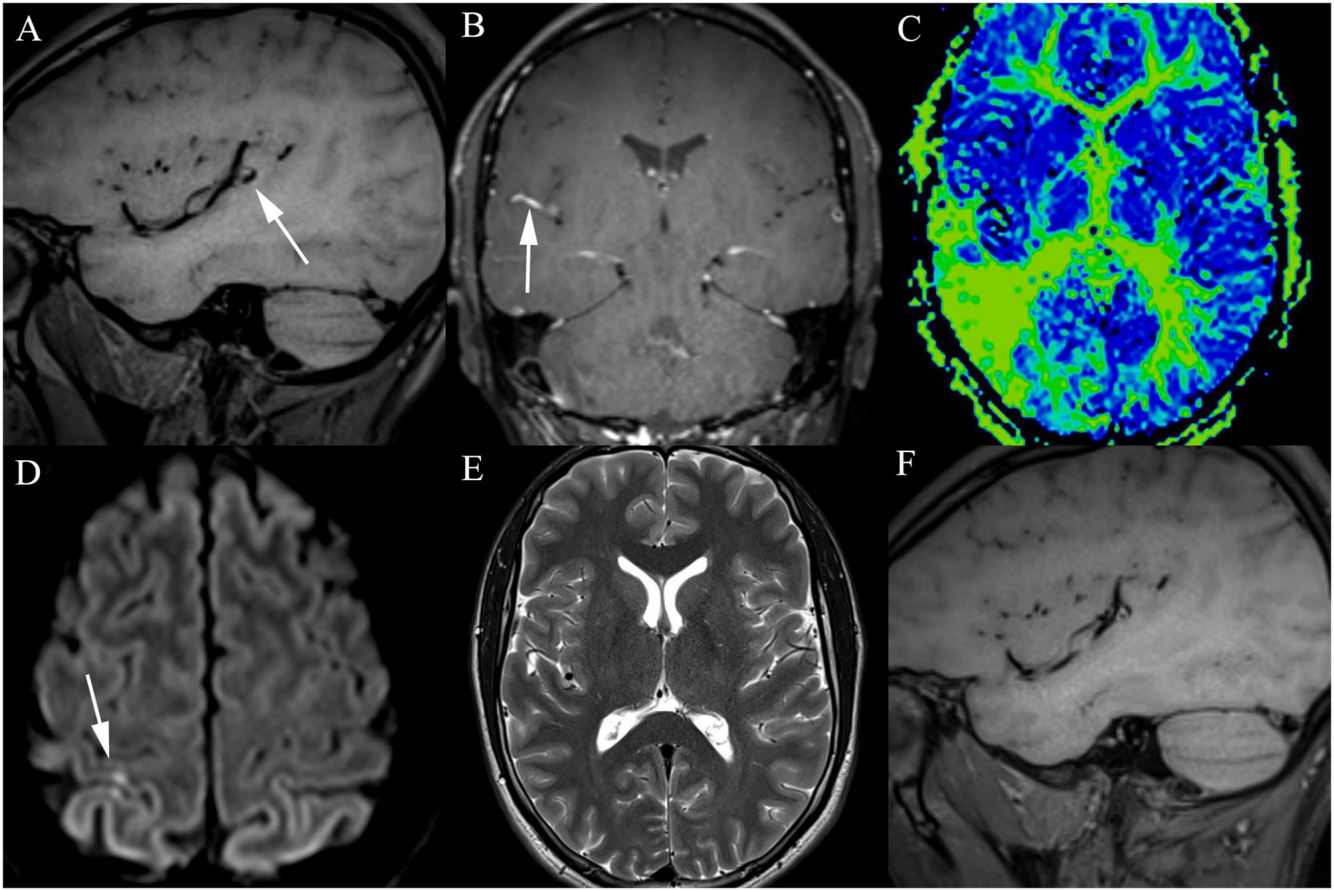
Postinterventional imaging findings: (A) T1-weighted image 6 months after flow diverter implantation showing hyperintense thrombotic material around the flow diverter and near-complete occlusion of the aneurysm. The initial severe stasis at the dome (Figure 2B) is depicted as a hypointense cap. (B) Slow flow in the side branch originating from the aneurysm wall is documented 6 months post intervention after changing to monotherapy with aspirin. (C) Perfusion deficit of the affected territory is visible on mean transit time reconstructions of the MRI perfusion. (D) Small ischemic lesions, located in the right superior parietal lobule, are seen on DWI. (E) One month after detection of the slow flow in the side branch of the aneurysm-wall, T2-weighted images did not reveal an infarction in areas with delayed perfusion. (F) As 12 month-follow-up MRIs reveal, the aneurysm is completely filled with a hyperintense thrombotic material, which means that full occlusion of the aneurysm was achieved.
